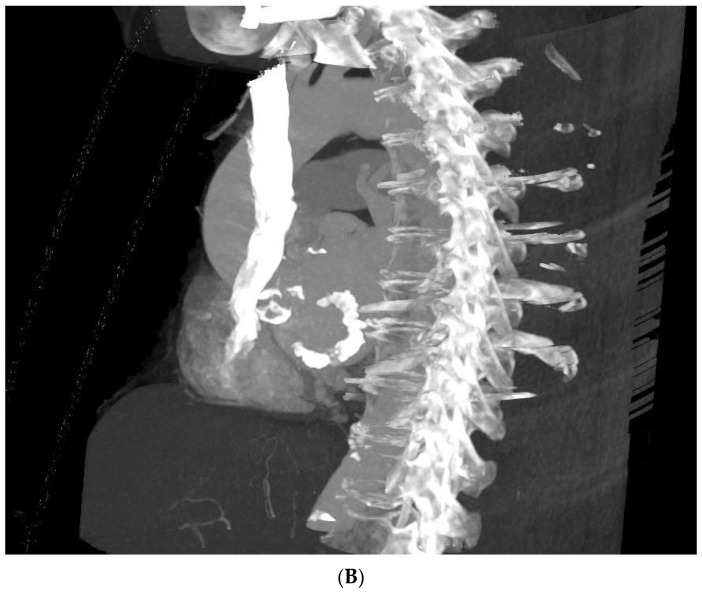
Computed Tomography showing extensive mitral annular calcification. (A) 3D recontruction of the calcified mitral annulus. (B) Computer tomography of the calcified mitral annulus.
